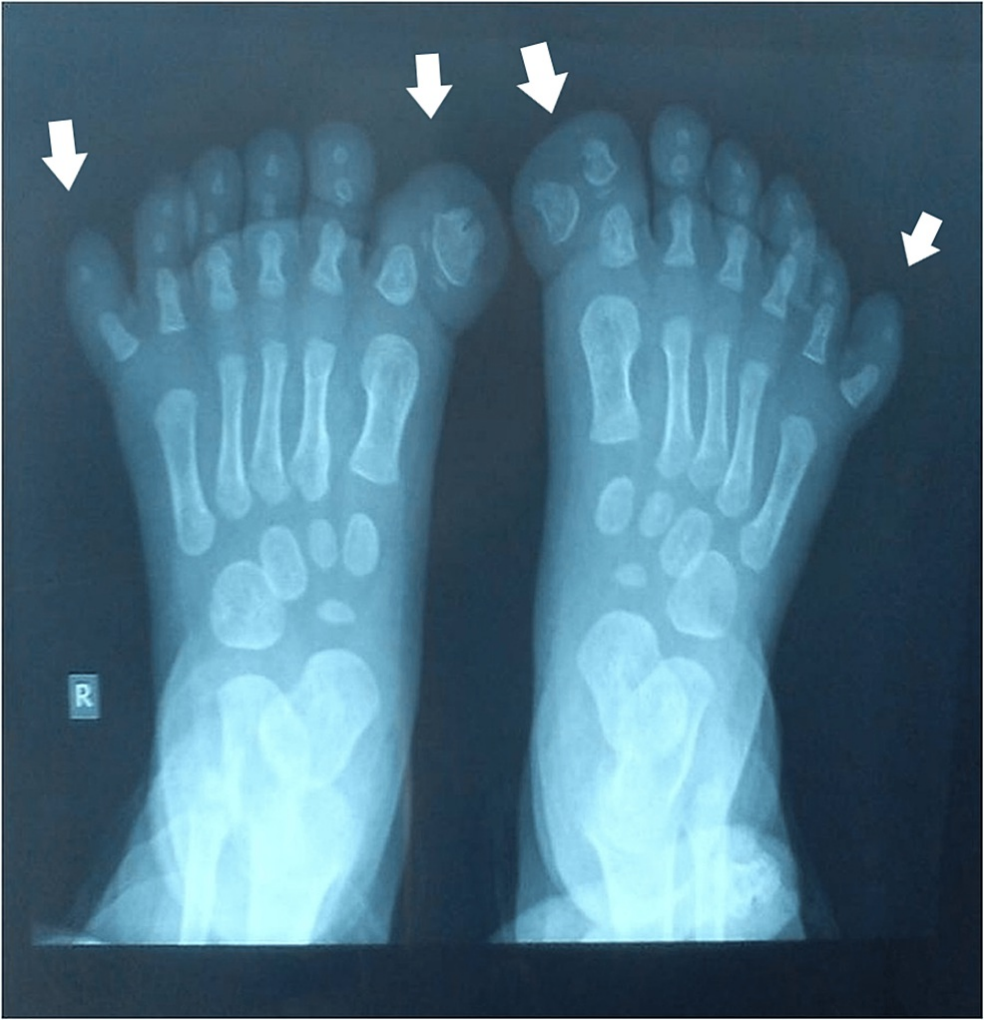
X-ray of the fourth patient's affected feet. X-ray of the feet with seven toes affecting both the medial and the lateral ray, with normal five metatarsals. Big toes that were duplicated were fused on the right side and had a varus position, while the nail was a broad one. On the left side, there was a single racket-type phalange and a developed two phalanges toe.
